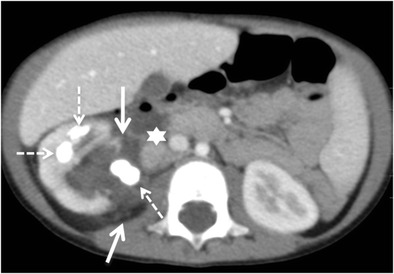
Diffuse XPN. Contrast-enhanced nephrogenic phase transverse CT shows multiple lithiasis in pelvis and calyces (*discontinuous arrows*), extrarenal extension (*white arrows*) and presence of adenopathy (*star*)
